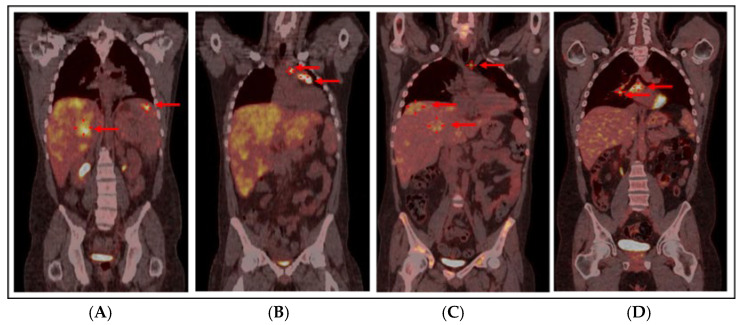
PET/CT scan at diagnosis: red arrows show extensive liver (SUV max 7.1) and splenic (SUV max 7) hypermetabolism (A) and lymphadenopathies in the left pre-tracheal (SUV max 8.5), pre-vascular (SUV max 8), and anterior mediastinal (SUV max 11.9) regions (B). (C) PET/CT scan after 3 cycles of ICE-21, before allo-HSCT: PMR with complete resolution of splenic hypermetabolic sites and mild reduction in mediastinal mass (32 mm, SUV max 4.6) and paratracheal and pre-vascular hypermetabolism (red arrow, SUV max 4.8), with persistent diffuse hepatic hypermetabolism (more pronounced in segments 4 and 7, as highlighted by red arrows, SUV max 4.8) in the context of hepatomegaly. (D) PET/CT scan 18 months after allo-HSCT: red arrows show an increase in the mediastinal mass (40 × 30 mm, SUV max 4.5) with the appearance of new hypermetabolism in the right paratracheal (SUV max 6.5), pre-carinal (SUV max 5.4), and sub-carinal (SUV max 7.2) regions and right hilar pulmonary (SUV max 6) lymphadenopathy, consistent with IPEH. Abbreviations: PET/CT: Positron Emission Tomography/Computerized Tomography, SUV: Standardized Uptake Value, ICE-21: Ifosfamide–Carboplatin–Etoposide, allo-HSCT: Allogeneic Hematopoietic Stem Cell Transplantation, PMR: Partial Metabolic Response, IPEH: Intravascular Papillary Endothelial Hyperplasia.
